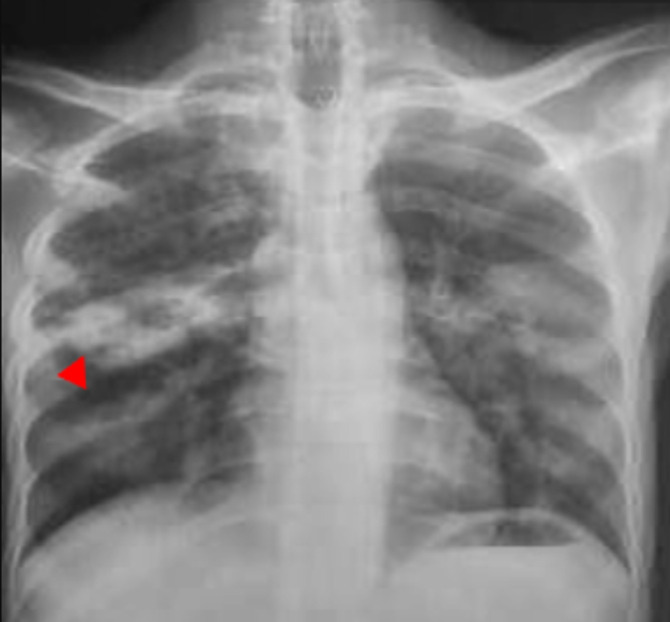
radiographie pulmonaire face montrant une image cavitaire avec une paroi opaque para-hilaire droite (tête de flèche), avant le traitement
